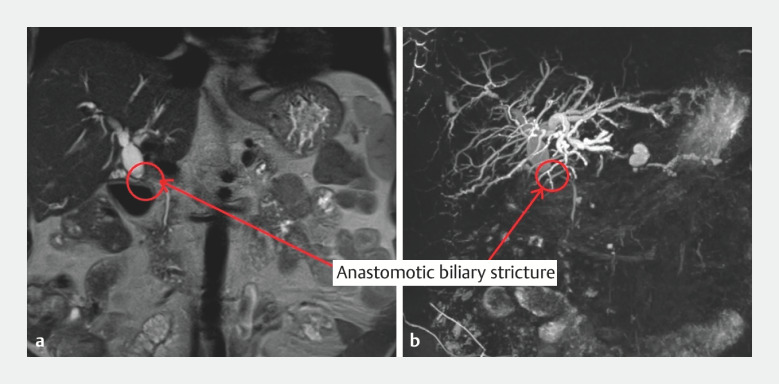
Coronal section magnetic resonance imaging (MRI) image showing a disparity in caliber between the recipient bile duct and the graft bile ducts.
